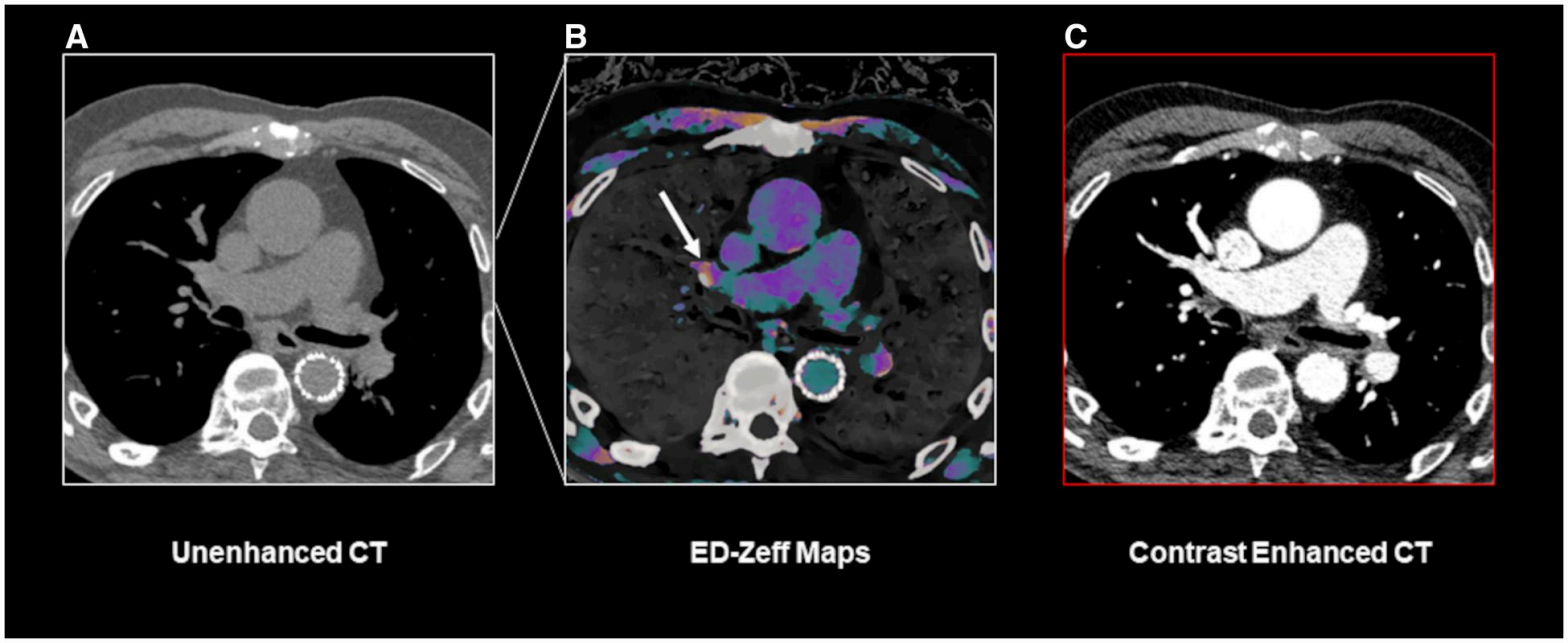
A false-positive case of PE identified using the ED-Zeff (electron density-Z-effective) map. An 82-year-old female patient presented to our department with chest pain. Unenhanced CT scan of the chest (A) shows no relevant vascular findings. ED-Zeff map (B), derived from the unenhanced CT scan, shows the presence of high signal spot within a right segmental artery (arrow). The subsequently performed CECT (contrast-enhanced CT) (C) shows no sign of pulmonary embolism.
